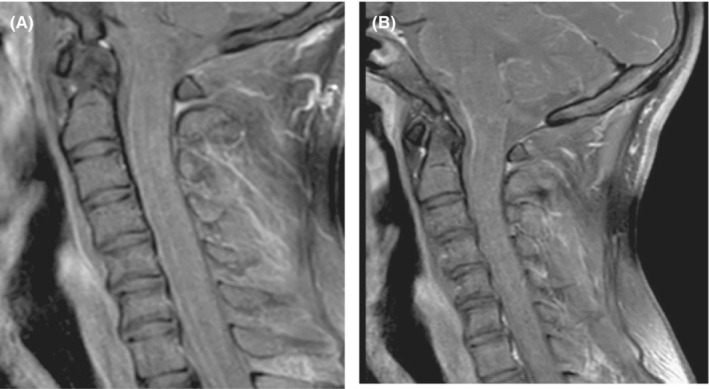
(A and B). MRI Spine Sagittal T1: post‐contrast showing dural thickening of cervical cord area.
